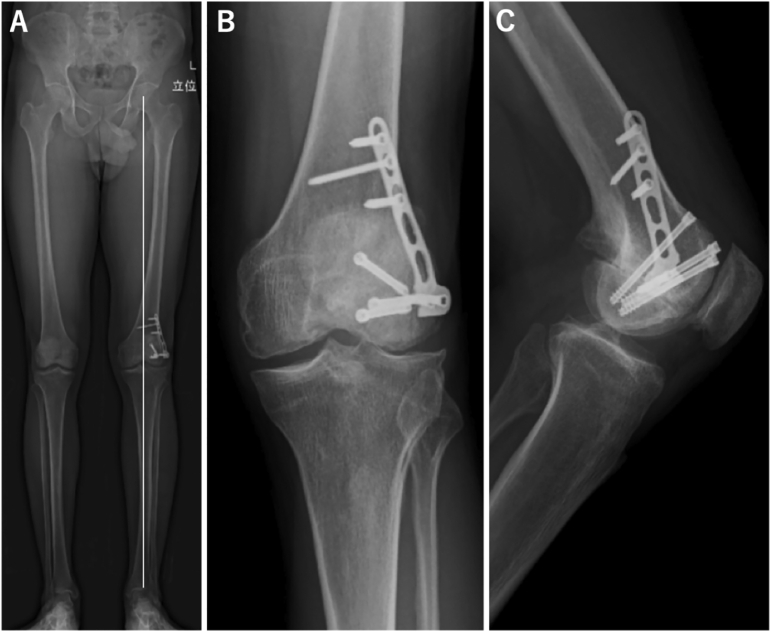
Follow up X-ray at 1 year. (A) Whole standing lower limb radiograph showed that the valgus deformity was corrected. (B) Anteroposterior view. (C) Lateral view.
